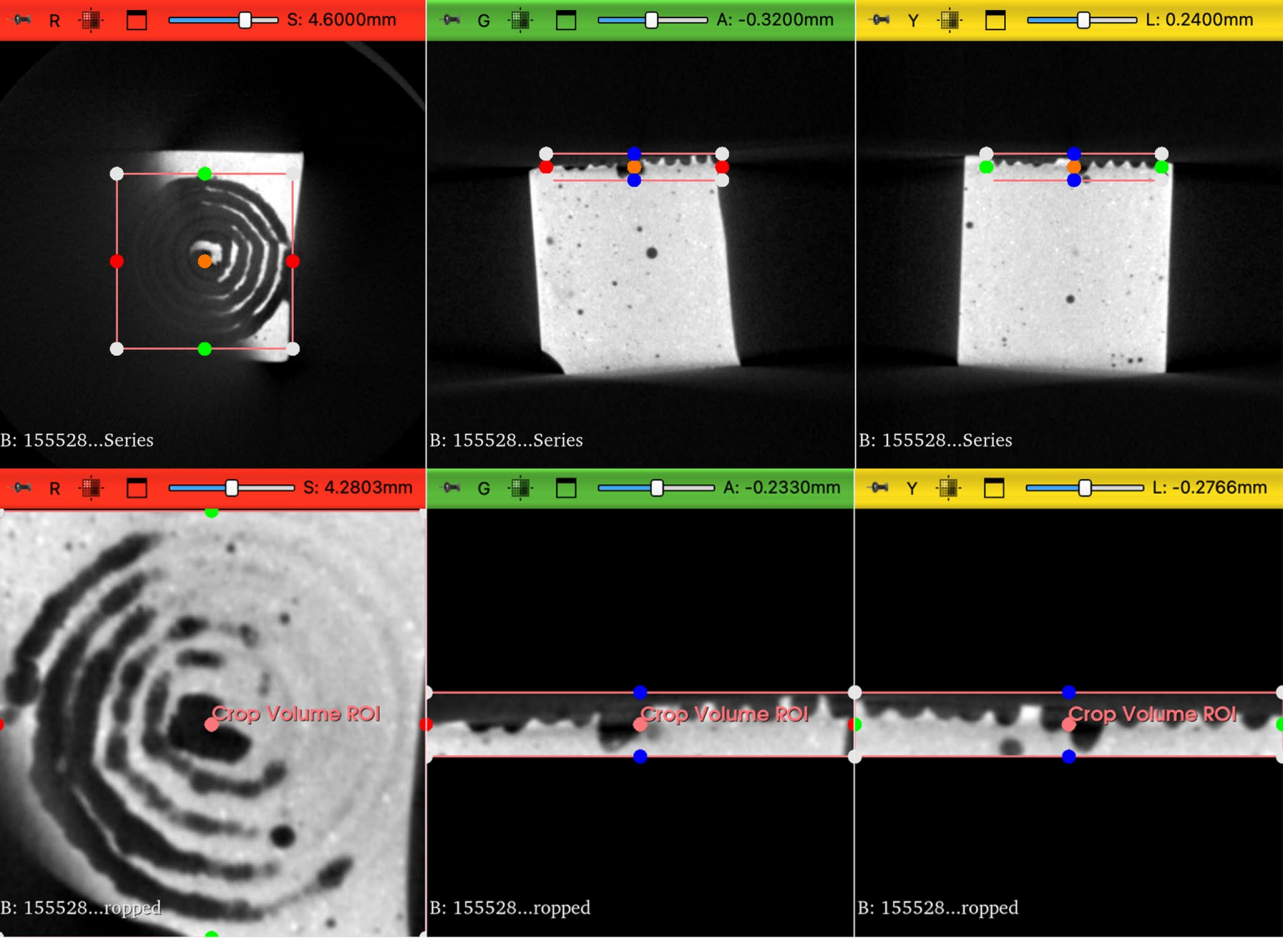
3DSlicer segmentation method to evaluate ablation volumes: stone segmentation from CT by selecting the region of interest (ROI)
